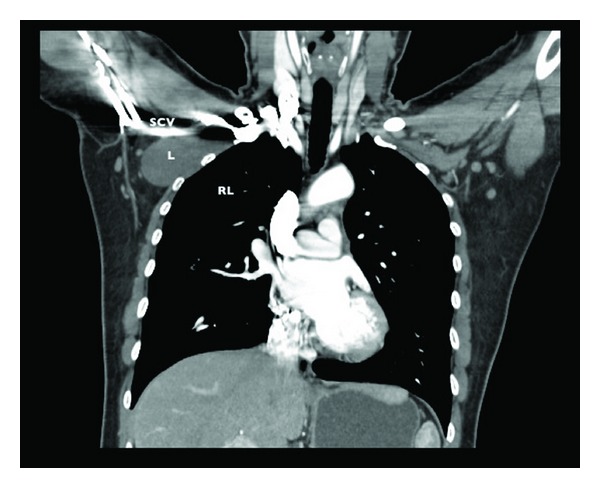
Chest CT with intravascular contrast, coronal view. L: lymphocele; RL: right lung; SCV: subclavian vessels.
